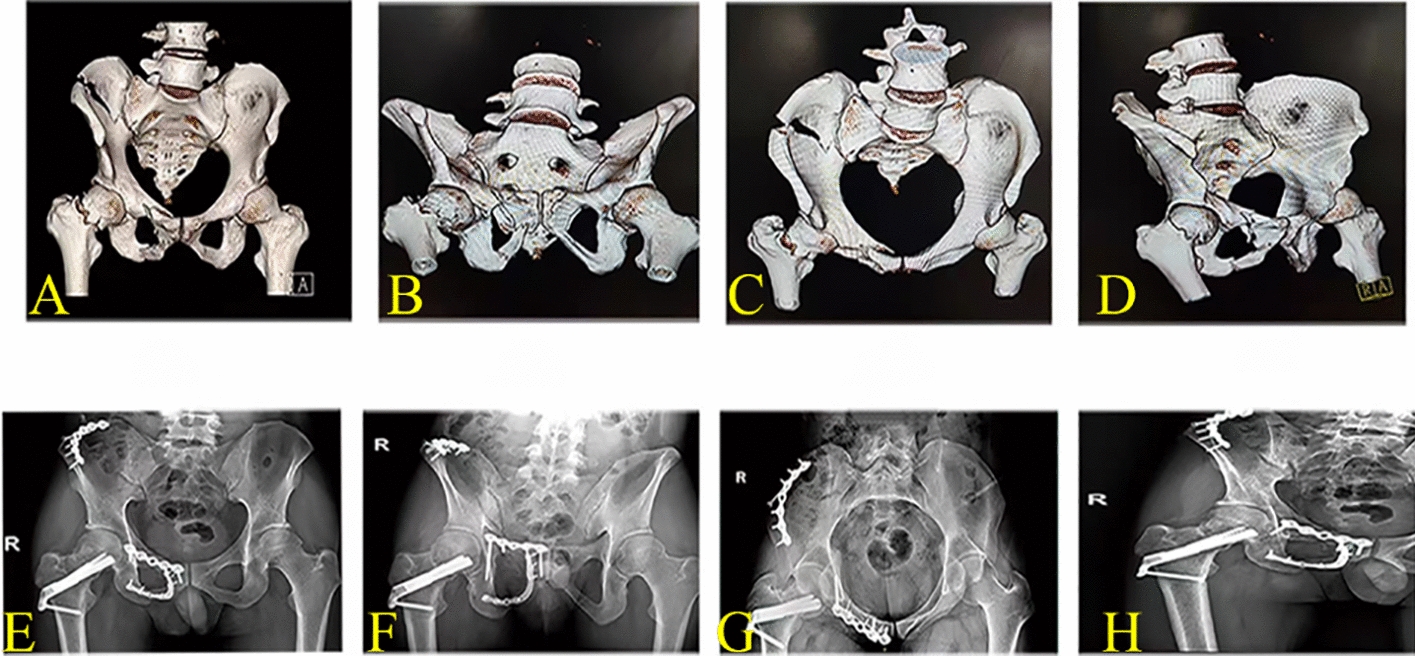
Typical case of the fixation of the superior and inferior ramus of pubis-ischium ramus fracture. A–D 3D reconstruction of preoperative CT images of the pelvis of a 32-year-old man with fractures of the right superior and inferior rami of the pubis-ischium ramus. E: anteroposterior view, F: outlet view, G: inlet view, H: obturator view, the postoperative X-ray of the male patient showing the male’s quality of fracture reduction was excellent
